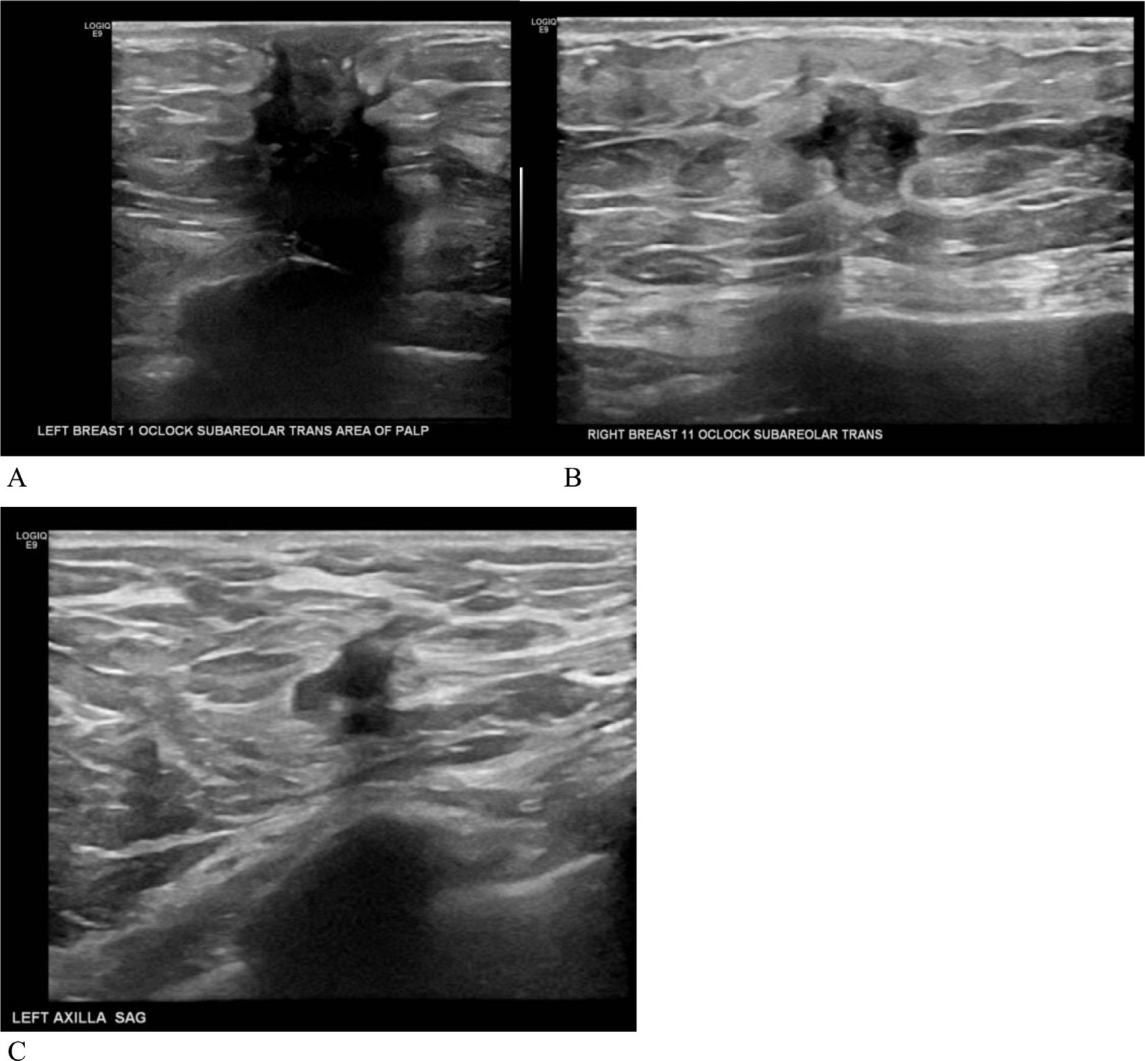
Grayscale targeted ultrasound images of the left breast (A) and right breast (B) and the left axilla (C) demonstrated bilateral, irregular masses and abnormal left axillary lymph node.
